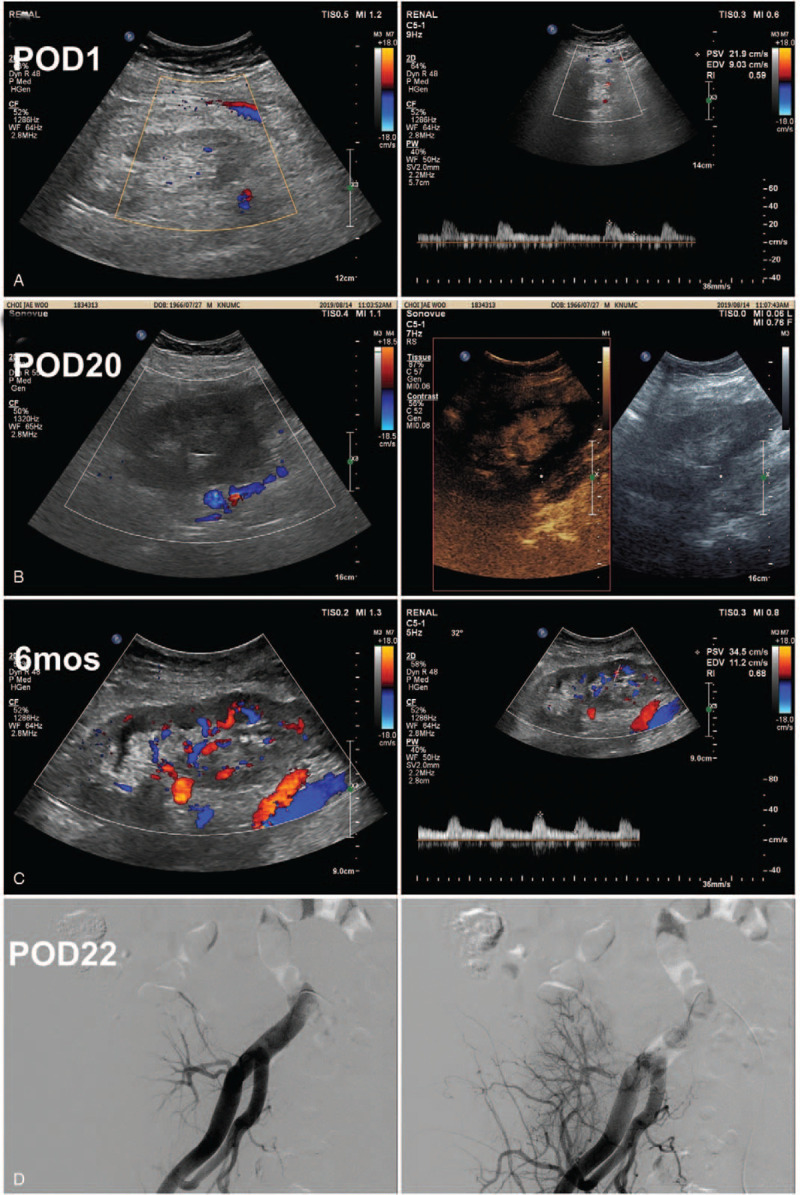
Changes of graft perfusion in graft Doppler sonography and graft angiography. (A) Decreased intrarenal perfusion with normal resistive index on postoperative day 1. (B) Decreased intrarenal perfusion, particularly in the cortical area, by allograft contrast-enhanced ultrasound on postoperative day 20. (C) Improved intrarenal perfusion with normal resistive index on the sixth postoperative month. (D) Right external iliac angiographic images on postoperative day 22. Graft renal arterial flow was good, but no cortical perfusion was noted.
